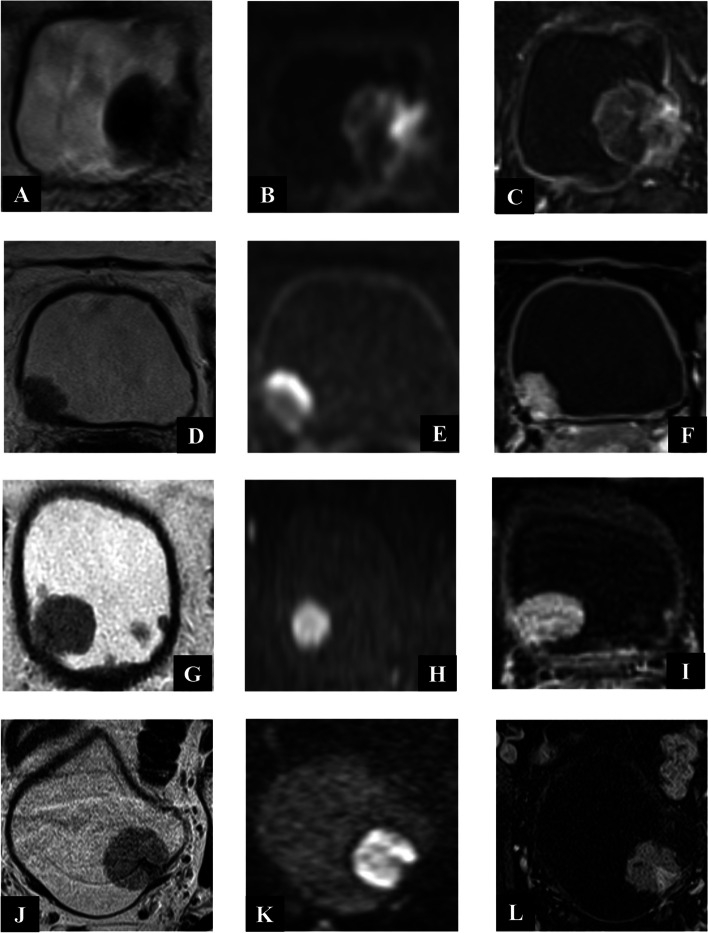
Examples of VI-RADS scores in our cases. VI-RADS 5 lesion on mp-MRI. A T2WI (B) DWI (C) DCE. VI-RADS 4 lesion on mp-MRI. D T2WI (E)DWI (F) DCE. VI-RADS 3 lesion on mp-MRI. G T2WI H DWI (I) DCE. VI-RADS 2 lesion on mp-MRI. J T2WI (K) DWI (L)DCE. Abbreviations: Vesical Imaging Reporting and Data System (VI-RADS), Multiparametric Magnetic resonance imaging (mp-MRI), T2-weighted imaging (T2WI), Diffusion-weighted imaging (DWI), Dynamic contrast-enhanced (DCE)
